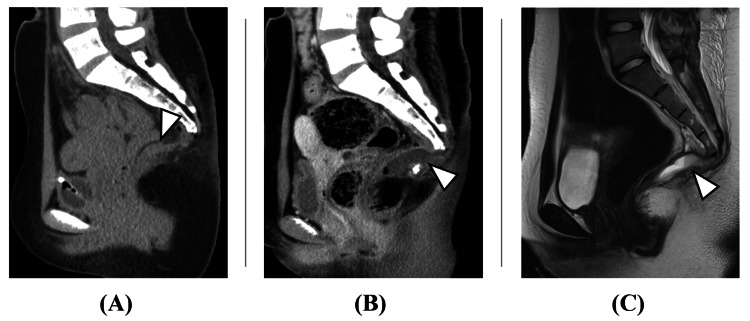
Post-initial surgery pelvic imaging. (A) Immediately post surgery (CT sagittal). (B) Two weeks post surgery (contrast CT sagittal). (C) Two weeks post surgery (MRI T2 sagittal). While immediate postoperative imaging showed cyst reduction with partial tumor remaining (arrowhead in Figure 5A), cyst fluid recurrence was observed two weeks after surgery (arrowheads in Figure 5B and Figure 5C).
